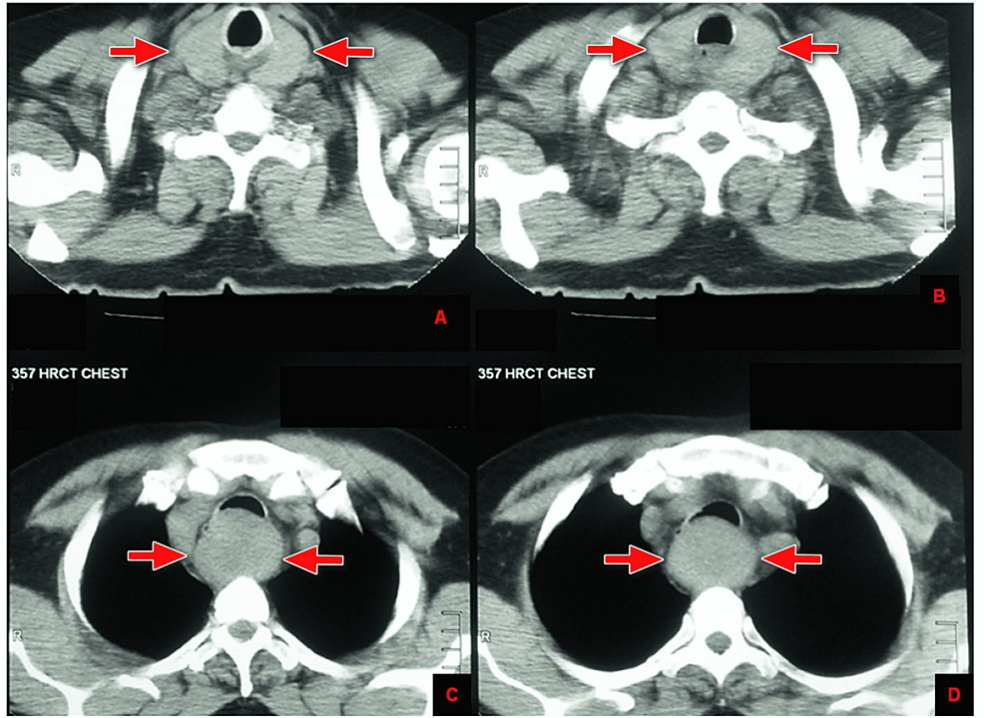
Axial HRCT of the chest with red arrows demonstrating a large smooth, soft tissue mass in the prevertebral location, extending from the cricoid post area (A and B) to the level of the aortic arch (C and D).
